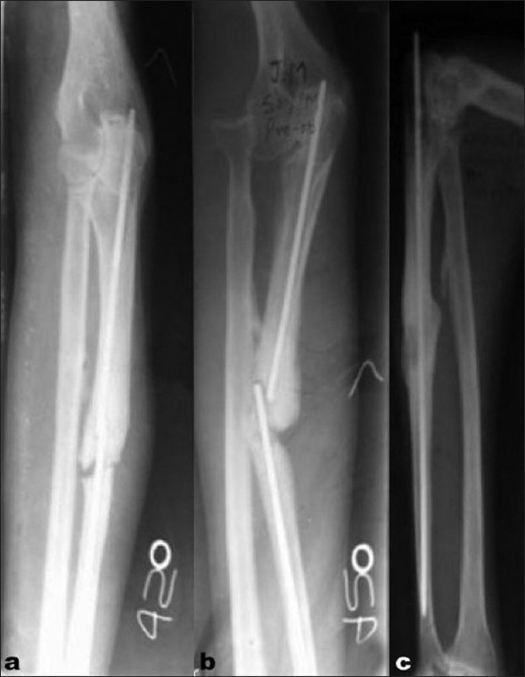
X-ray of left forearm with elbow lateral (a) and anteroposterior view (b) of a case of 2-year-old neglected Monteggia fracture dislocation with ununited ulna and broken implant *in situ* (intramedullary nail). Lateral view (c) shows radial head excision and fracture fixation with intramedullary nail and interposed tricorticocancellous strut bone graft incorporation at 24 weeks follow-up
